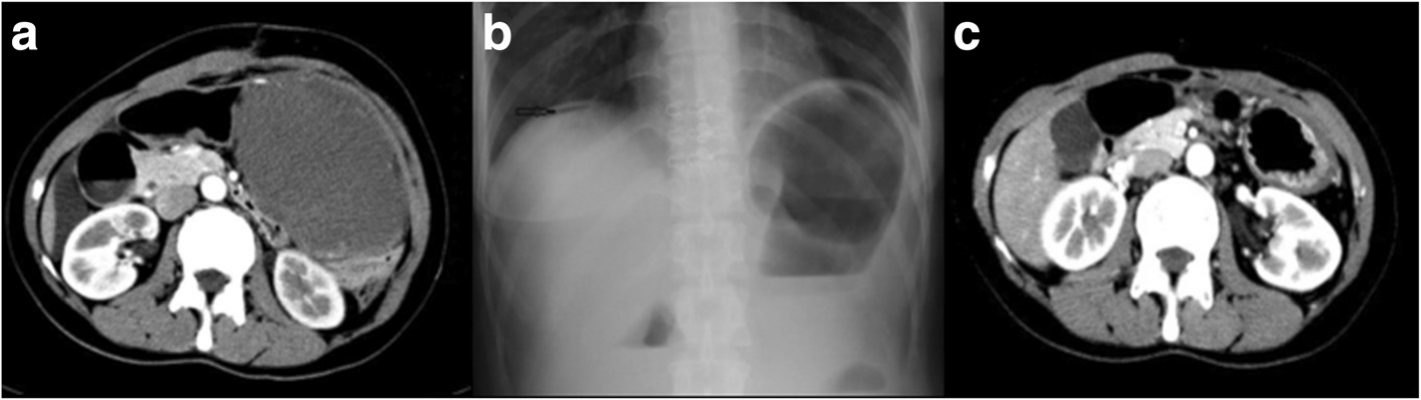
The progression of the intra-abdominal desmoid in patient 1. a CT scan before DOX/DTIC therapy showing a large mass in the abdomen in October 2012. b X-ray imaging displayed the shadow of free gas (as indicated by the *arrow*) below the right diaphragm. c Follow-up CT scan in February 2016
